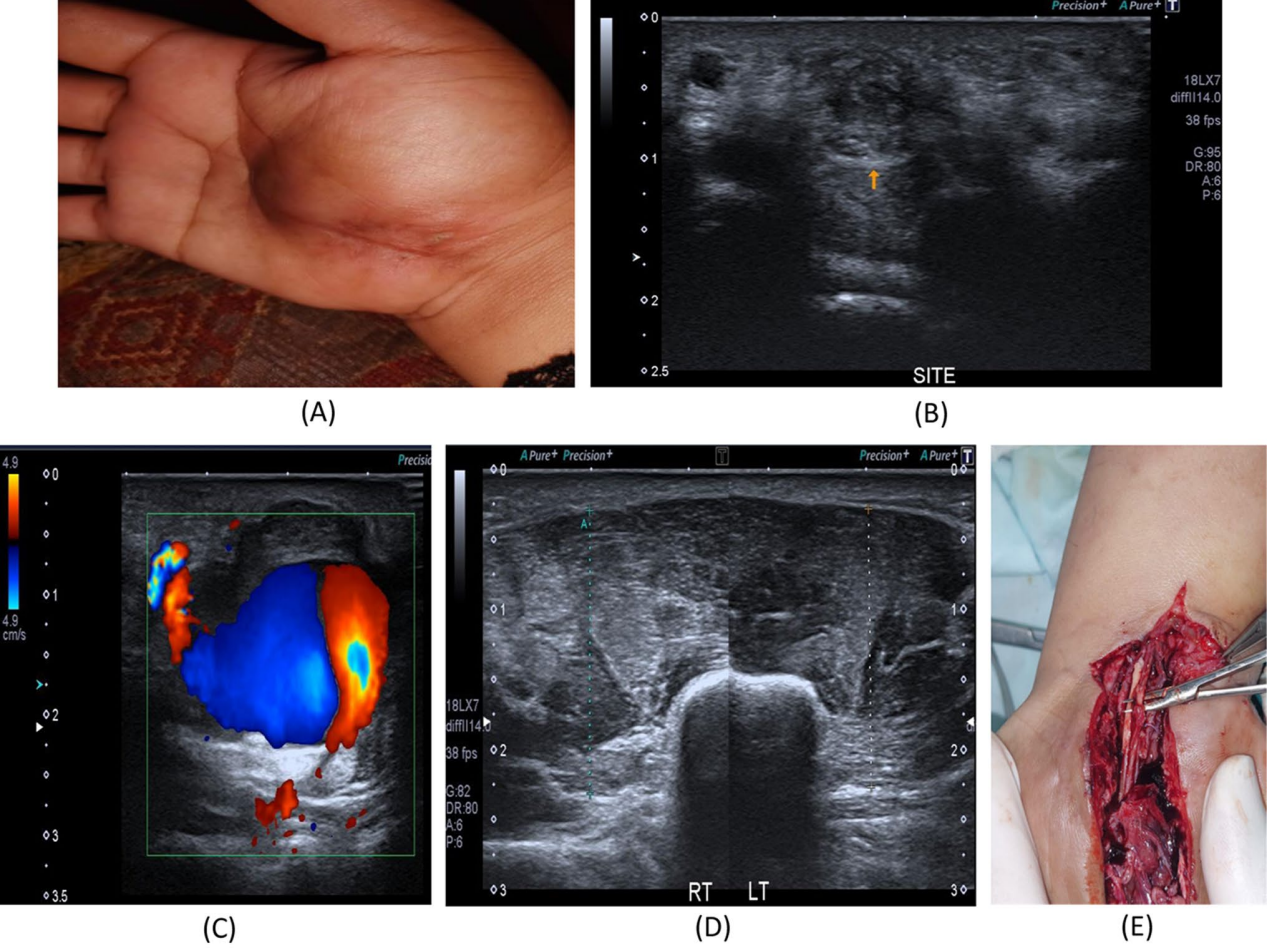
26-year- old female with a history of right distal radial fracture in July 2021 treated, After the cast removal, numbness and tingling along the radial aspect of the palm with loss of sensation of the middle finger and newly developed progressive increased thenar swelling. A Patient’s hand with prominent swelling of the thenar eminence and mid-palmar incision scar. B B-mode image, transverse axis, reveals loss of normal sonographic appearance of the left median nerve at the carpal tunnel with related hypoechoic superficial scar tissue, suggesting partial nerve injury. C Color Doppler mode image reveals pseudo-aneurysm; Pepsi sign with an area of luminal thrombosis. D The B-mode image of the thenar muscles, increased echogenicity of the left thenar muscles as compared to the right side with rather preserved girth. E Intraoperative image revealed complete injury of two of the sensory branches of the median nerve
